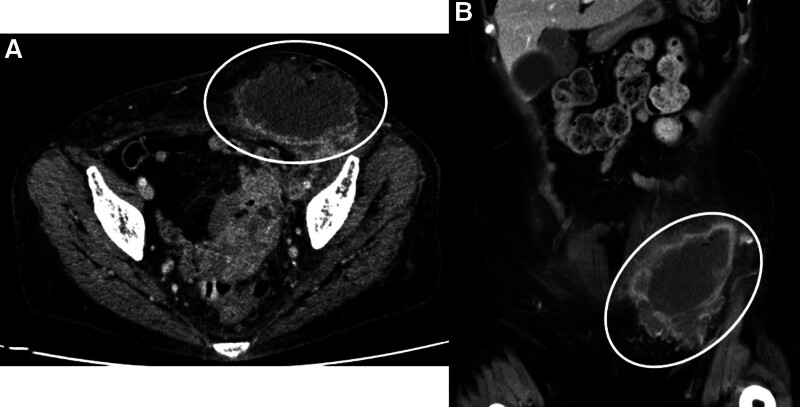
Contrast-enhanced computed tomography showed large abscess formation with gas in the left inguinal region extending from the left retroperitoneum. (A) Coronal image (circle). (B) Sagittal image (circle).
